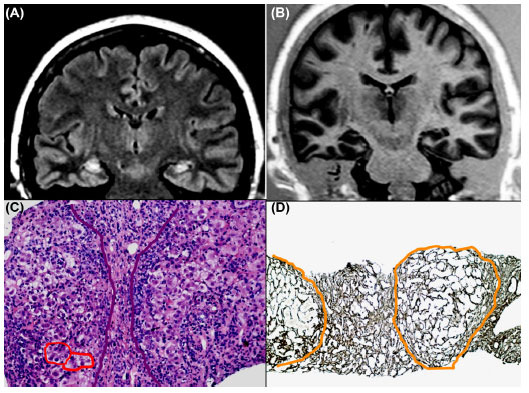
A 58-year-old woman with drug-resistant TLE and memory deficits. Brain MRI demonstrates bilateral hippocampal hyperintensities on T2-weighted images (A) with prominent left hippocampal atrophy (B). She also has an undifferentiated connective tissue disease and chronic auto-immune hepatitis, stage III. On liver biopsy, note the severe necro-inflammatory activity (red circles), fibrous septa and hepatocellular rosettes (Emat-Eos.20X) (C) and subversion of the acinar structure with segregation of noduliform areas of parenchyma (Gomori 10X) (D). Steroid therapy, in addition to ASMs, ameliorated control of epileptic seizures and resolved mild cognitive deficits. Inflammatory and immunological factors might contribute to epileptogenesis in TLE.
